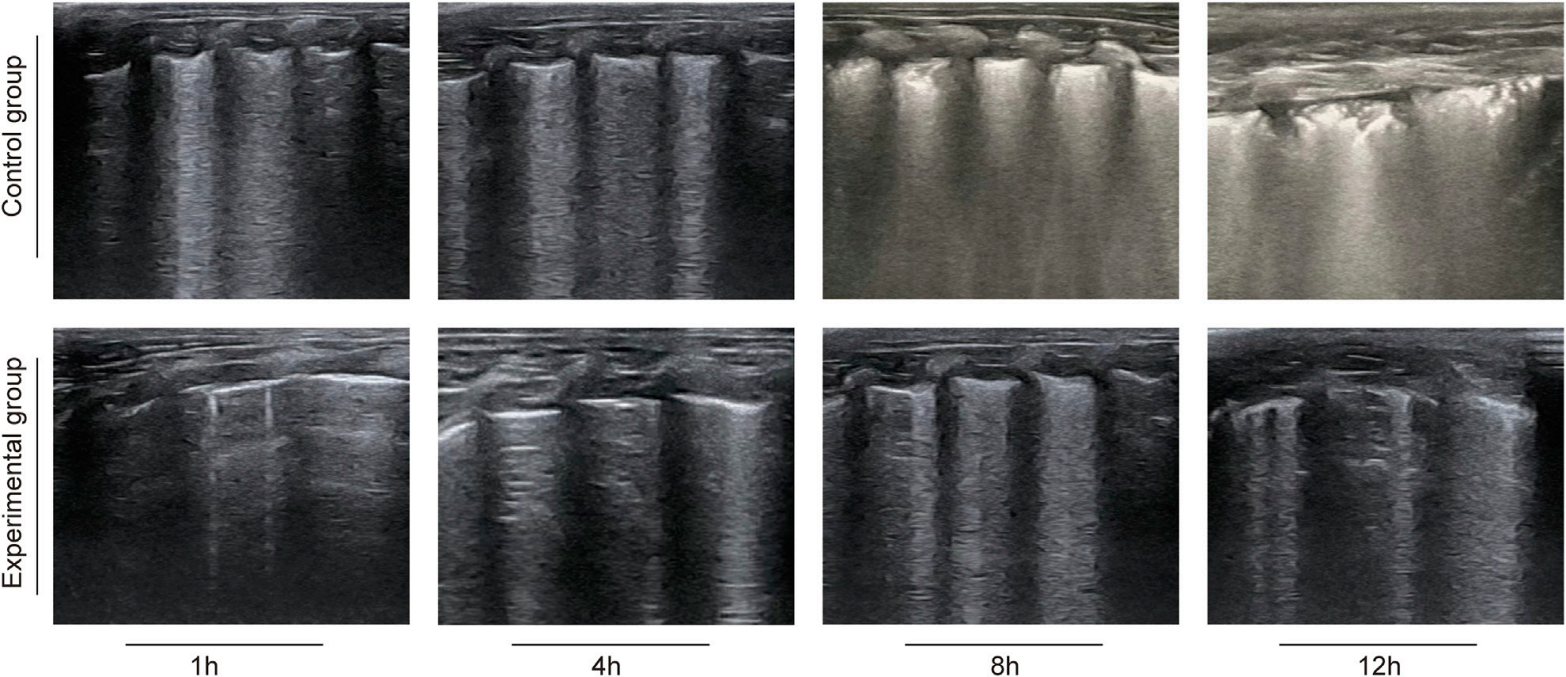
Representative lung ultrasound images in rabbits with oleic acid–induced acute lung injury.
